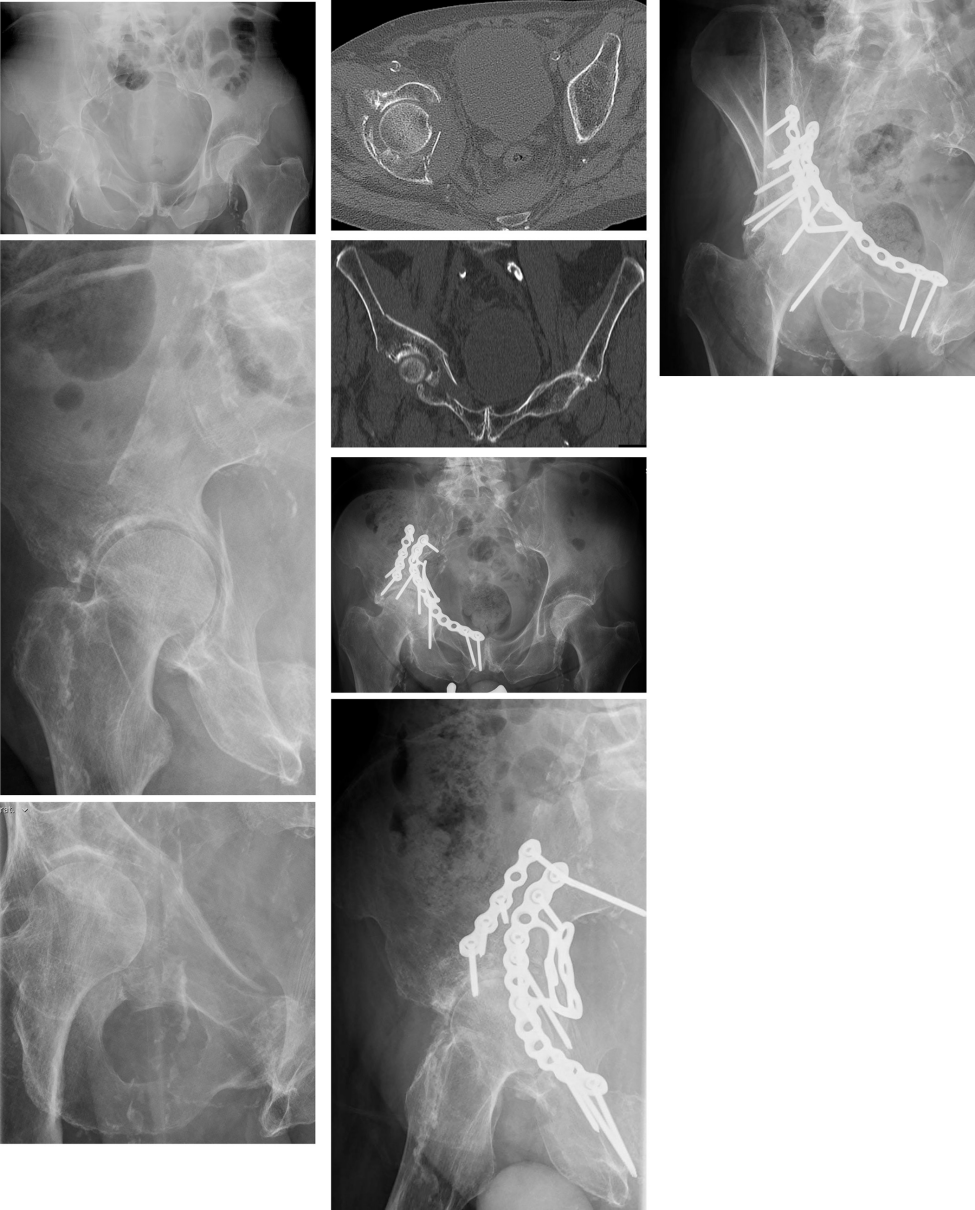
Sixty-eight year old man with anterior column and posterior hemitransverse fracture after alcoholic fall. (a) Pelvic ap overview. (b) Ala view. (c) Obturator view. (d) Axial CT cut through the acetabulum. The displaced quadrilateral plate is visible. (e) Coronal CT through the acetabulum. The quadrilateral plate is displaced medially togehter with femoral head. (f) Pelvicap overview one year after ORIF. The quadrilateral plate has been reduced and secured in its position with a suprapectineal quadrilateral surface plate. (g) Ala view. (h) Obturator view. The patient shows good mobility with a rolling walking frame.
